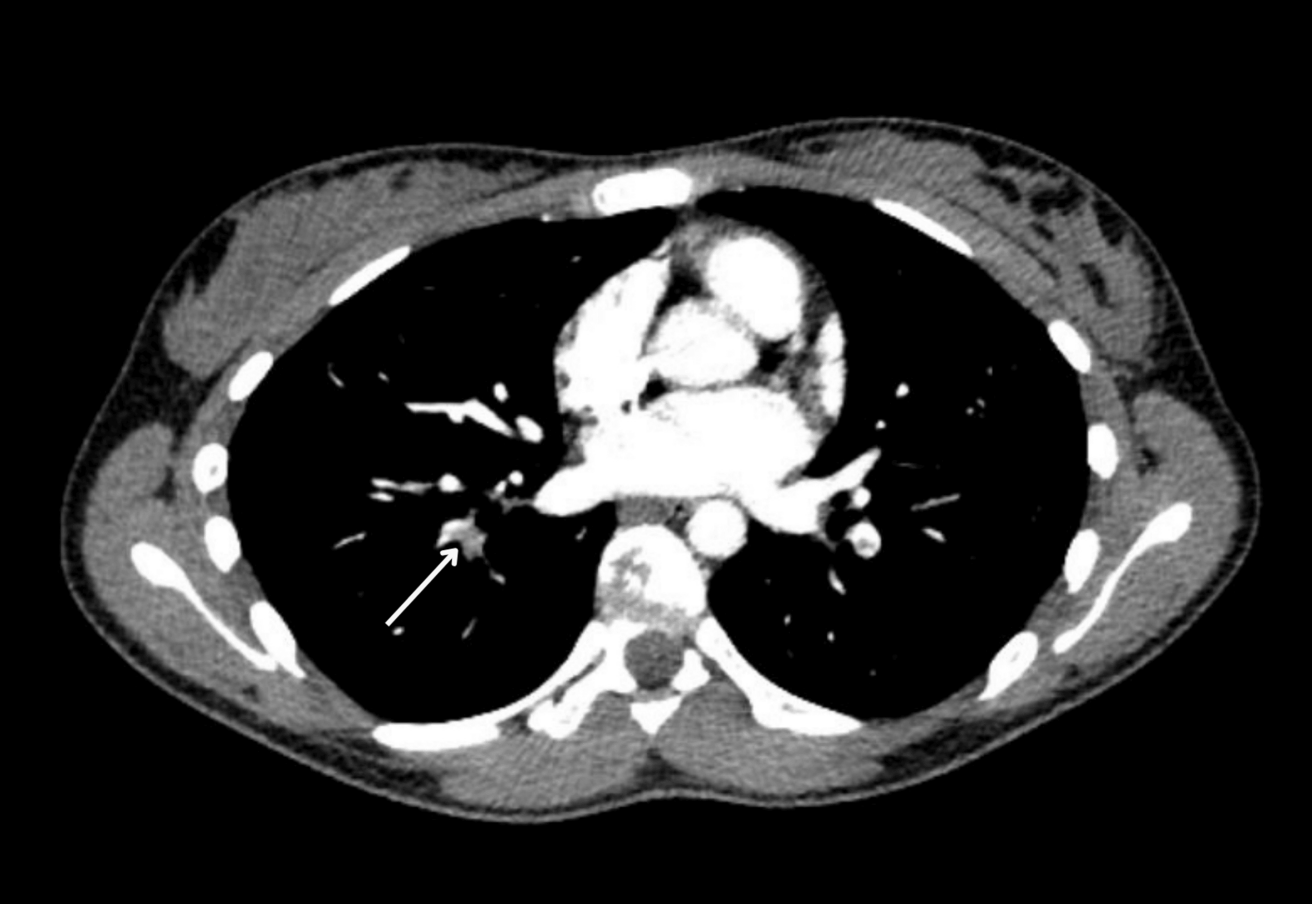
Axial CT pulmonary angiography (CTPA) image from Case 1 demonstrating a filling defect within a segmental pulmonary artery branch supplying the right lower lung lobe (arrow)
